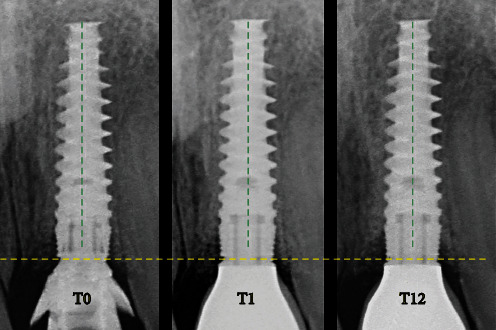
MBL measurement on calibrated intraoral radiographs at T0, T1, and T12.
